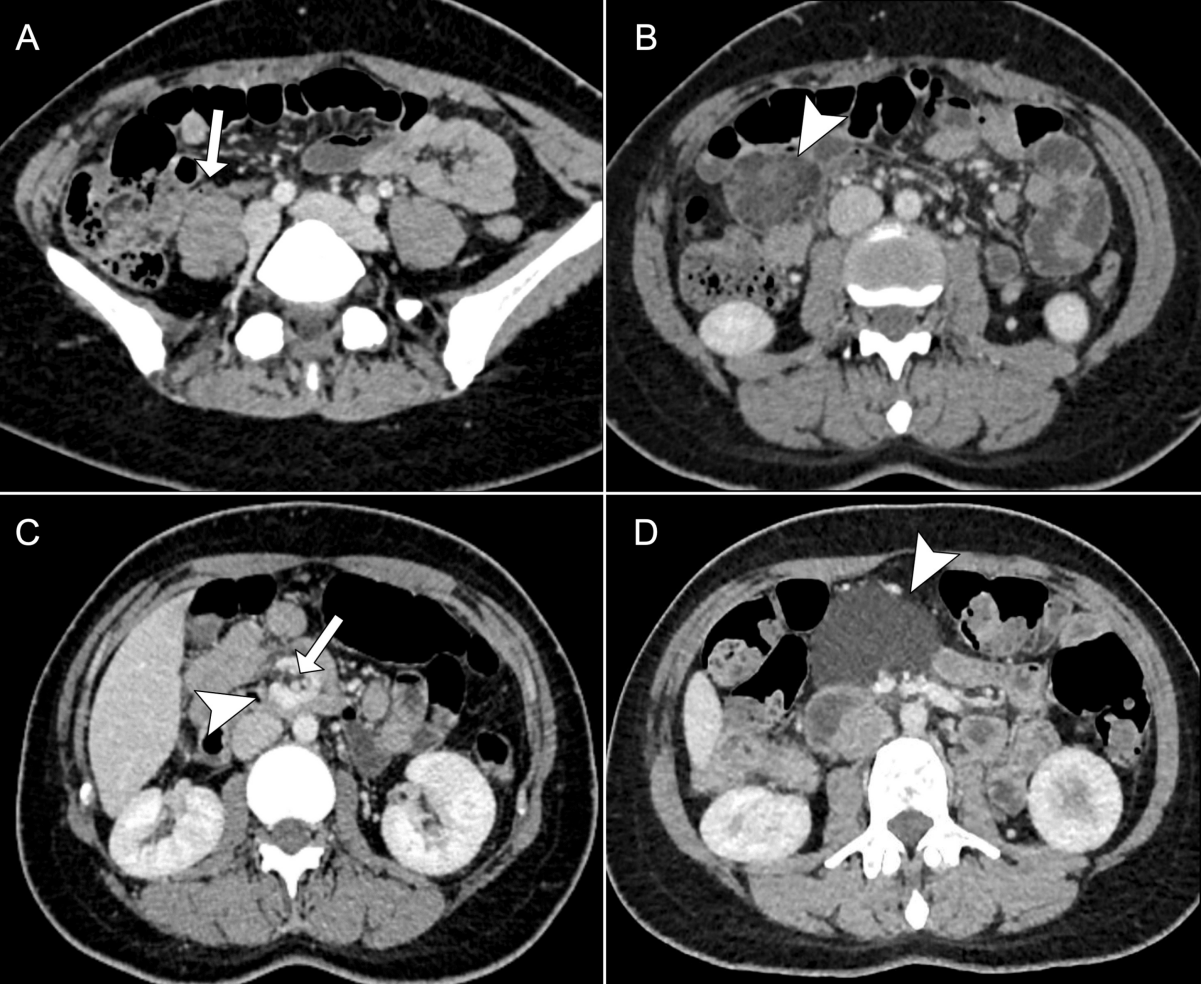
Current (A, B, and C) and previous (D) abdominal CECT in the portal venous phase, axial reconstructions, 1-mm slice thickness A: Axial image shows a normal cecal appendix (arrow). B: Mesenteric heterogeneous mass contacting jejunum in the right flank (arrowhead) with lobulated, well-defined margin, having fat and fluid densities (Hounsfield units range from -55 to +20), peripheral and internal septations with mild enhancement. There was no small bowel obstruction. C: Relationship between superior mesenteric artery (arrow) and superior mesenteric vein (arrowhead) with "whirl sign" suggesting torsion. D: Appearance of lesion on previous CECT (four months before), simple cystic mass with no noticeable walls (arrowhead), more centrally located and without the vascular "whirl sign" (not shown). CECT: contrast-enhanced computed tomography
